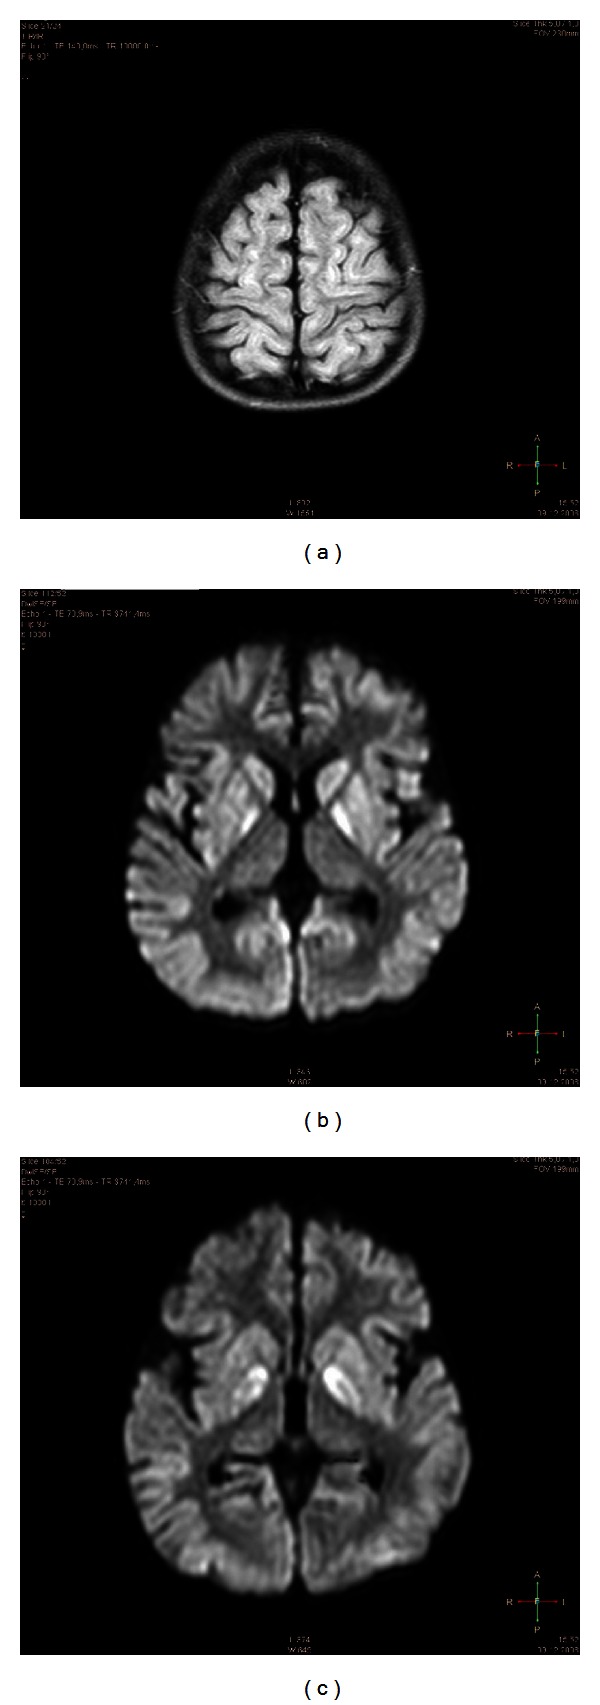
(a), (b), and (c) Brain MRI of the patient (L.B.) 2 weeks after cardiac arrest. Note signs of severe global ischemia in cortical structures as evidenced by (a) signal hyperintensity of gyri in almost entire cortex (FLAIR sequences) and basal ganglia (b), including caudate nucleus, pallidum, and putamen (c) (FLAIR DWI sequences with contrast media).
